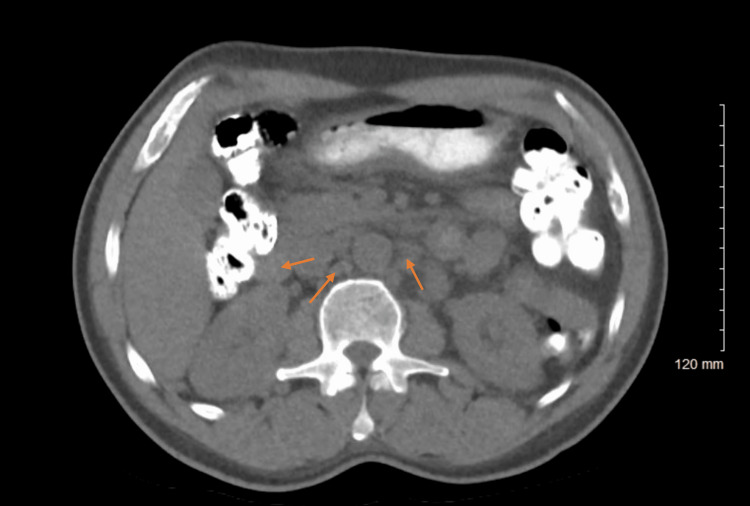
CT scan of the abdomen and pelvis demonstrating mesenteric and retroperitoneal lymphadenopathy (arrows).
